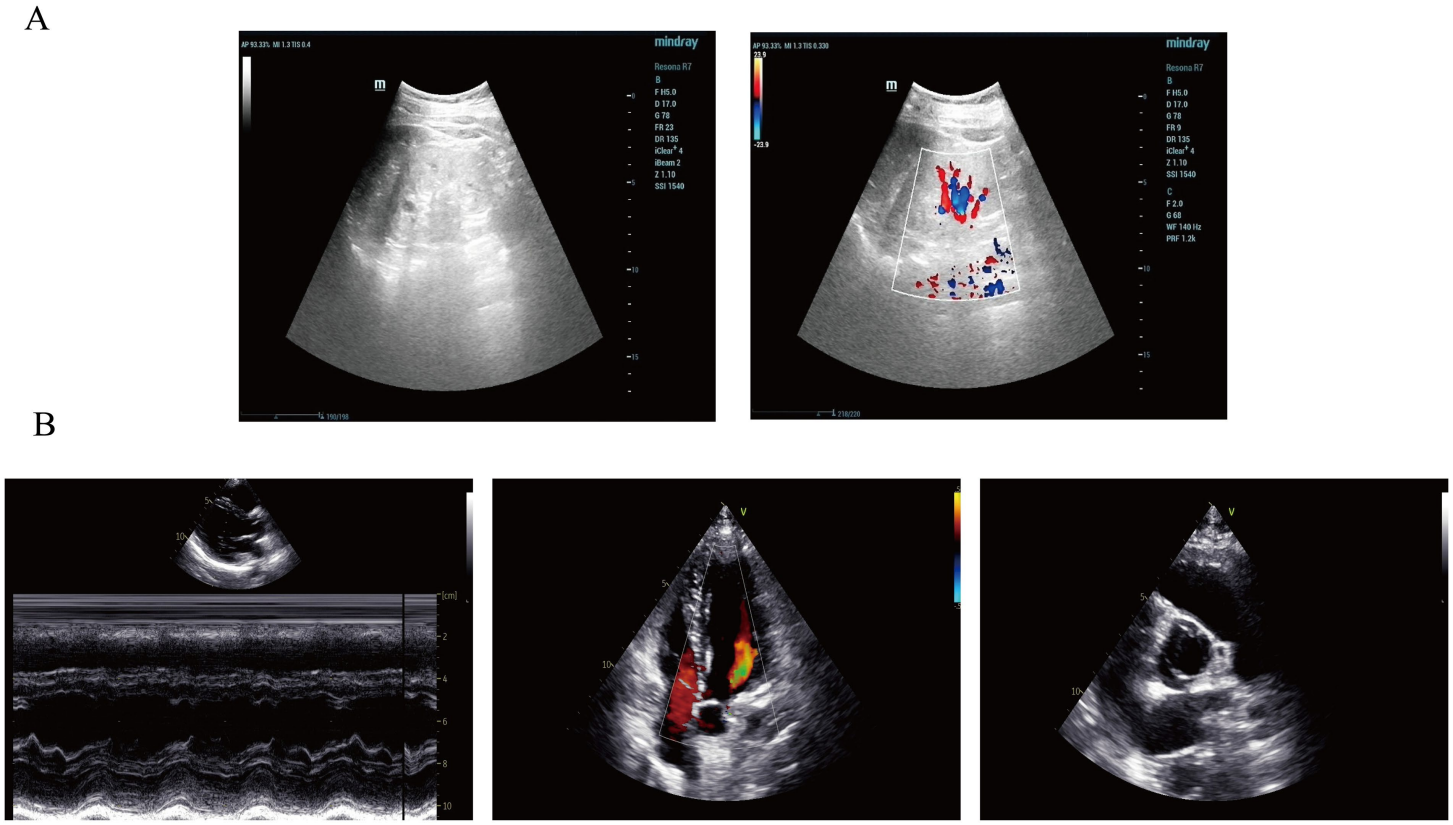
Imaging findings of the patient in this case report. (A) Renal ultrasonography revealed multiple anechoic lesions in the renal cortex. In the right kidney, the largest cyst was located at the lower pole, measuring 0.8 × 0.5 cm, with well-defined margins and good internal echogenicity. In the left kidney, multiple cortical cysts were observed, with the largest measuring 1.0 × 0.8 cm in the mid-region, showing clear margins and good posterior acoustic enhancement. These findings are consistent with bilateral renal cysts. (B) Color Doppler echocardiography (CDE) demonstrated normal sizes of all cardiac chambers, with no thickening of the interventricular septum or left ventricular wall, and preserved wall motion. The aortic valve showed fusion of the left and right coronary cusps, arranged in a right–left orientation with a commissural angle of approximately 160°. A fusion ridge was visible between the fused cusps, with good valve opening and a commissural slit, indicating a congenital bicuspid aortic valve (right–left, Type I). The morphology and structure of the remaining valves appeared normal.
